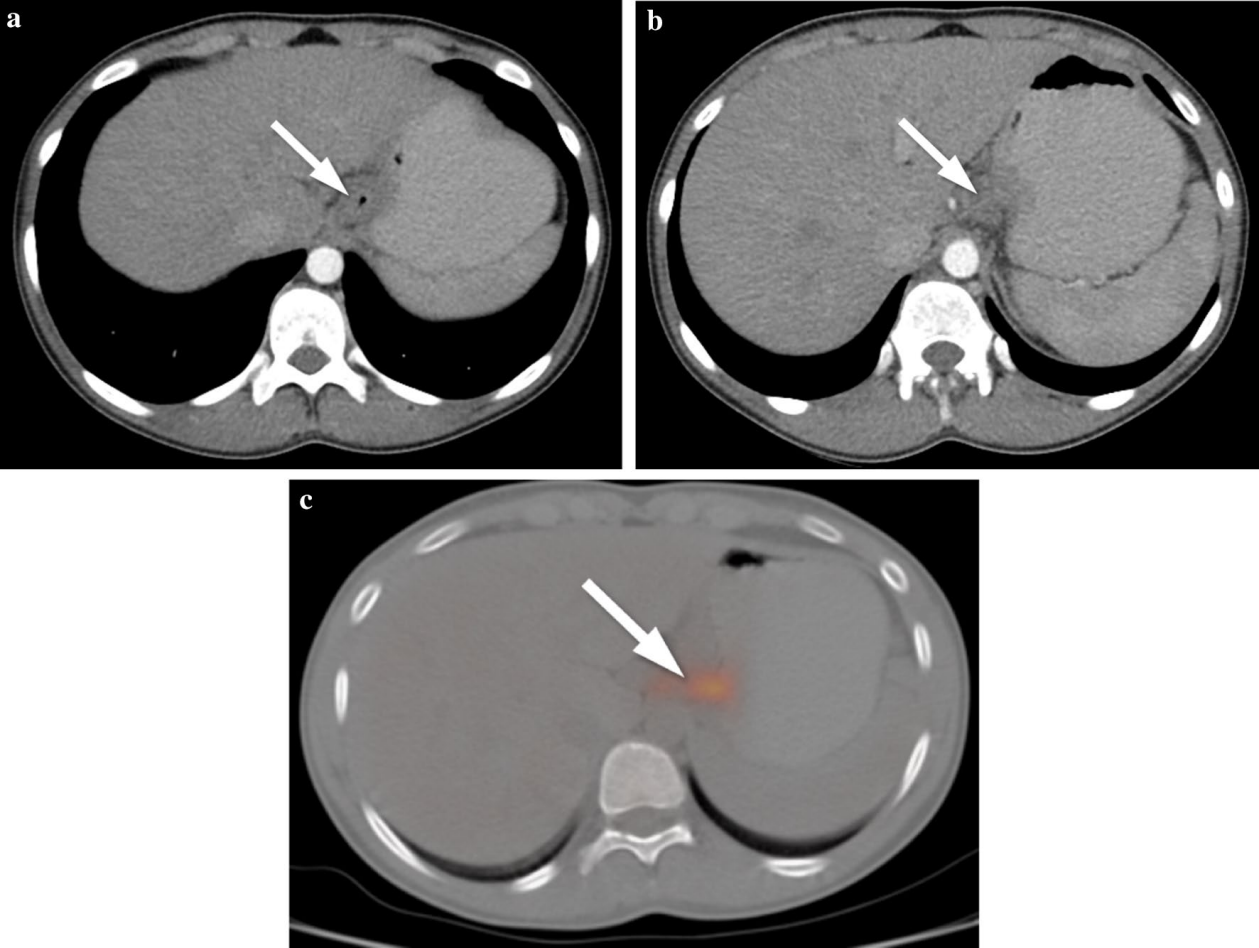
a–c A 14-year-old girl with esophagus adenocarcinoma. a, b Axial contrast-enhanced CT images show diffuse hypodense wall thickening at the distal part of the esophagus (arrows). c PET/CT image shows focal hypermetabolic activity at the distal part of the esophagus corresponding to the mass seen on CT
